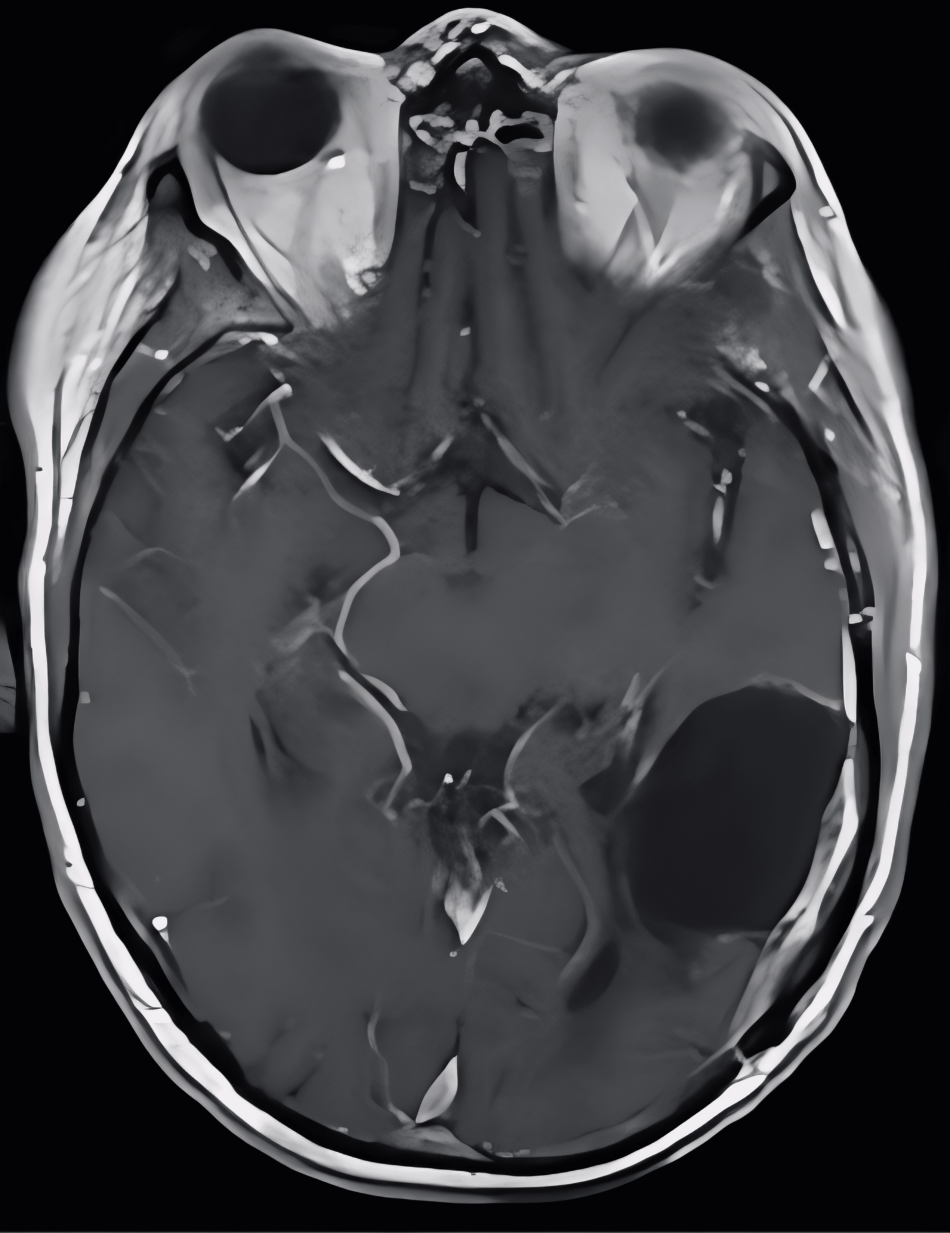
Postoperative MRI of the brain with and without contrast Initial postoperative MRI of the brain shows no significant residual tumor present.
